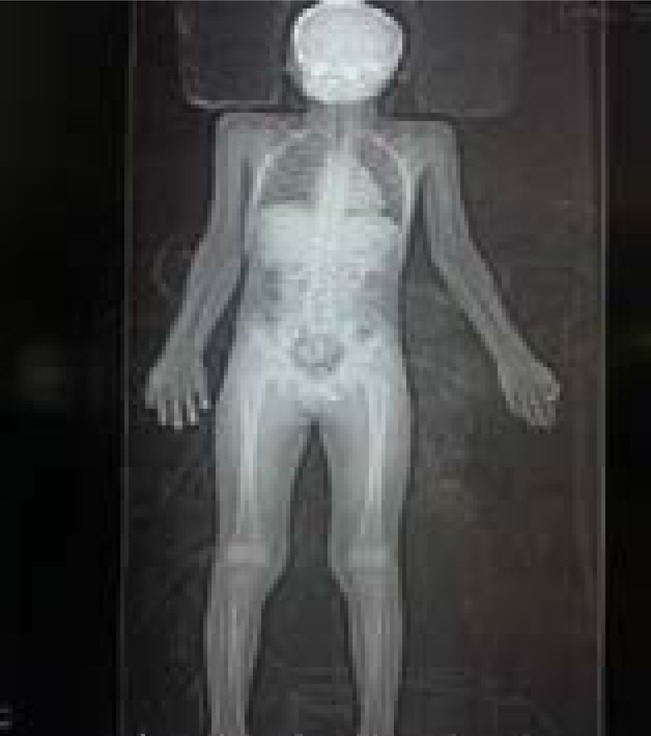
A low dose whole body x-ray (LODOX) performed in the trauma resuscitation room.
